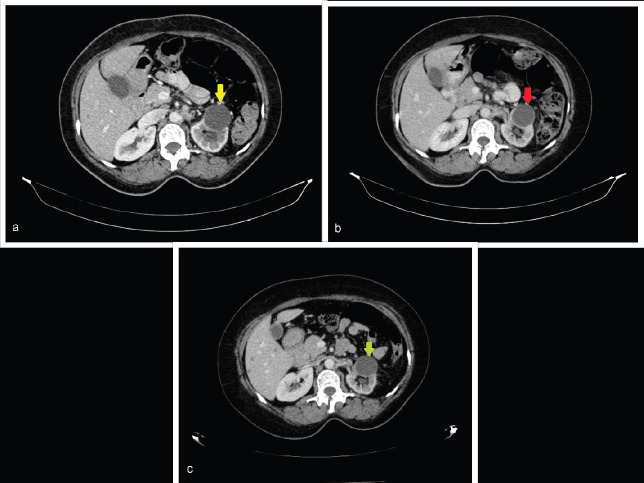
Representative axial cut images of the abdominopelvic contrast-enhanced computed tomography (CECT) scan. (a): CECT performed in July 2020 showing a mild interval decrease in left kidney hypo-enhancing lesion from 41 × 38 mm to 33 × 29 mm (yellow arrow) and similarly a decrease in size of the retroperitoneal node from 20 × 16 mm to 19 × 10 mm. (b): CECT performed in December 2020 showing a decrease in size in the left kidney hypo-enhancing lesion from 33 × 29 mm to 28 × 26 mm (red arrow). (c): CECT performed in September 2021 showing significant reduction in size of renal mass from 41 × 38 mm to 28 × 24 mm (green arrow) and retroperitoneal nodes from 20 × 16 mm to 16 × 9 mm.
